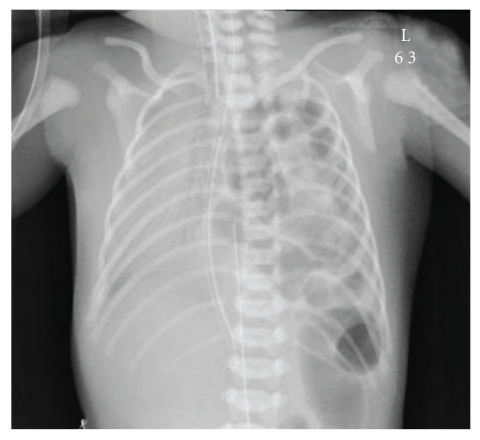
Chest X-ray shows left-sided congenital diaphragmatic hernia with herniating loops of large bowel into the right hemithorax.
